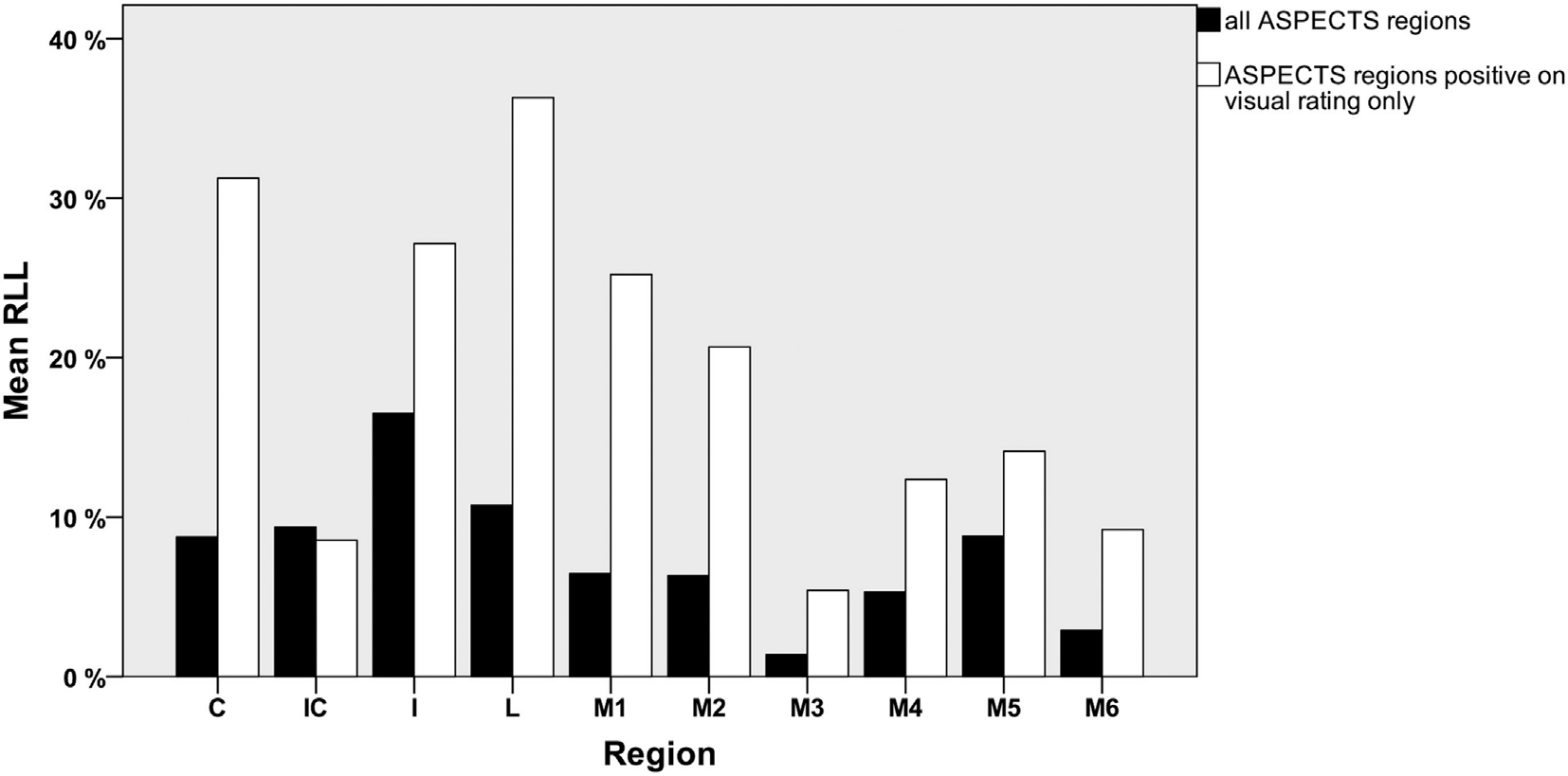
Mean relative lesion load (RLL) *per* ASPECTS region for all patients (black) and mean RLL for visually positive regions only (white).
